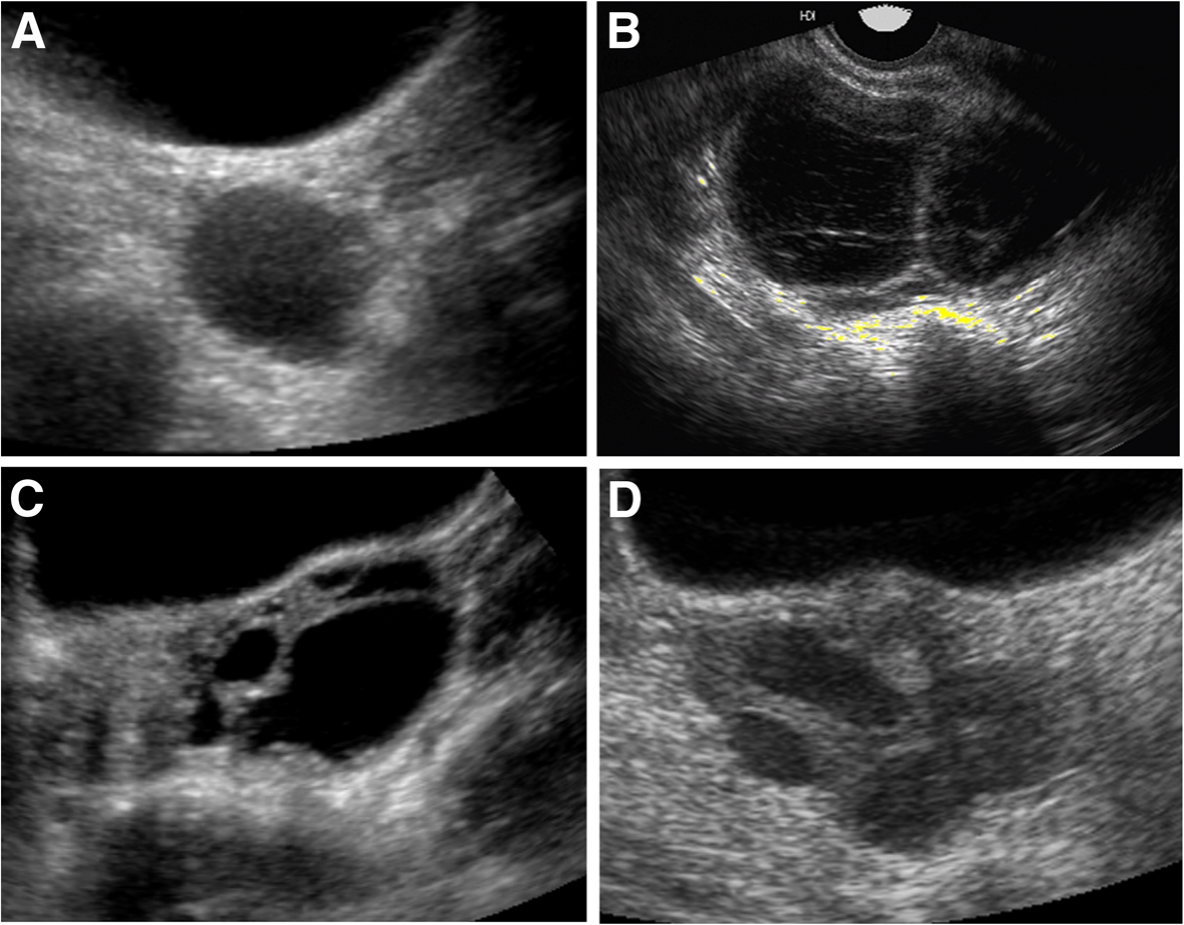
Representative pelvic ultrasound images. a Solitary cyst, measuring 4 cm in diameter. b Septated ovarian cyst, measuring 4 cm in diameter. c Multicystic adnexal mass, measuring 6 cm in diameter. d Multiple cysts involving the right ovary, the largest measuring 3 cm in diameter
